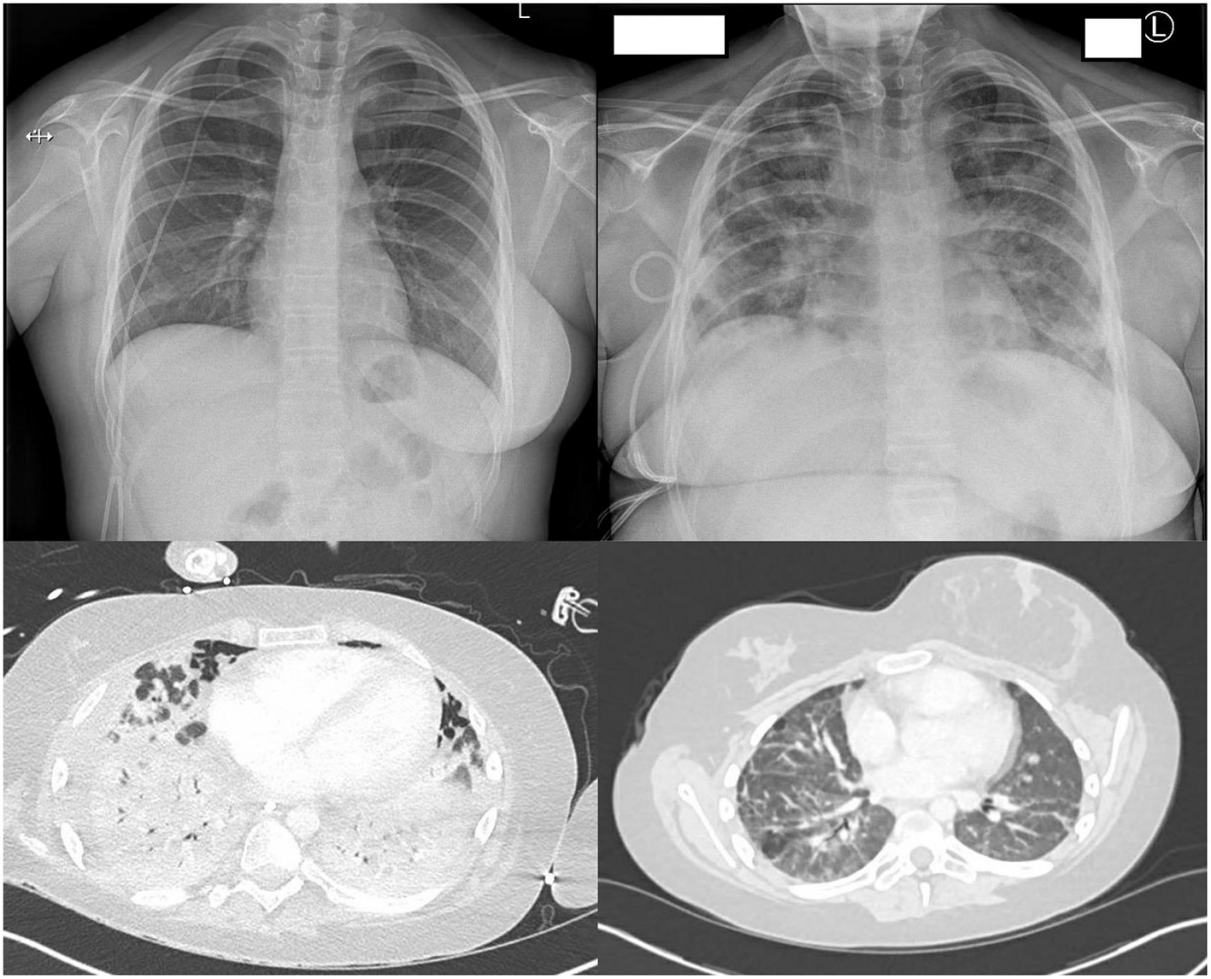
Chest radiology during admission. Top left: Chest X-ray at baseline (Day −1). Top right: Chest X-ray at ARDS development (Day +10). Bottom left: CT thorax post-tracheostomy (Day +47) showing extensive inflammation fitting with COVID-19. Bottom right: CT thorax 3 months post discharge showing residual lung changes.
